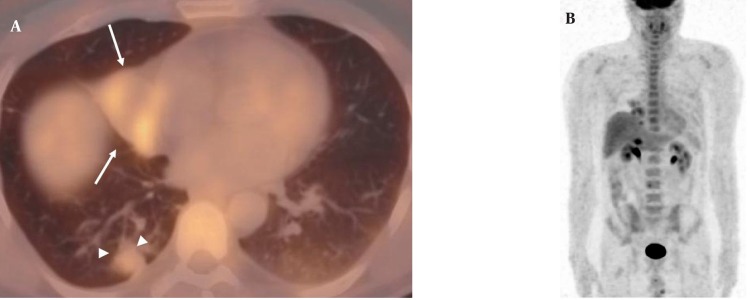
A, Axial PET-CT fusion image demonstrates that both the mass (arrows) and nodules (arrowheads) show FDG uptake with a SUVmax value of 2.9 and 2.5; B, Coronal MIP PET image shows no extrapulmonary involvement. (MIP: maximum intensity projection, SUVmax: maximum standardized uptake value, PET-CT: Positron emission tomography-computed tomography, FDG: Fluorodeoxyglucose)
